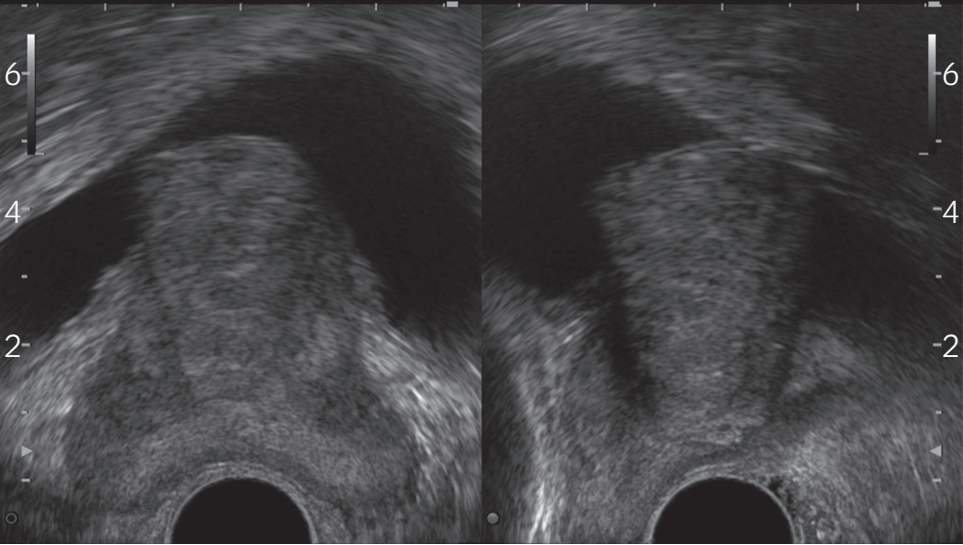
Prostate ultrasonography showed a marked mid‐lobe hypertrophy that extended toward bladder lumen.
